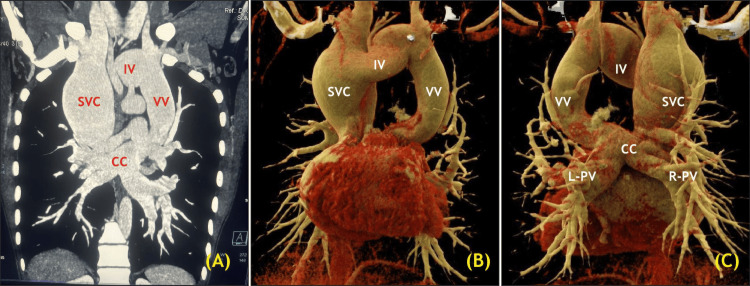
Preoperative CT angiography showing (A) the CC ascending into the VV; (B) 3D reconstruction (anterior view); and (C) 3D reconstruction (posterior view). SVC: Superior vena cava; IV: Innominate vein; CC: Common chamber; L-PV: Left pulmonary veins; R-PV: Right pulmonary veins; CT: Computed tomography. Image credit: Dr. Sambhunath Das
